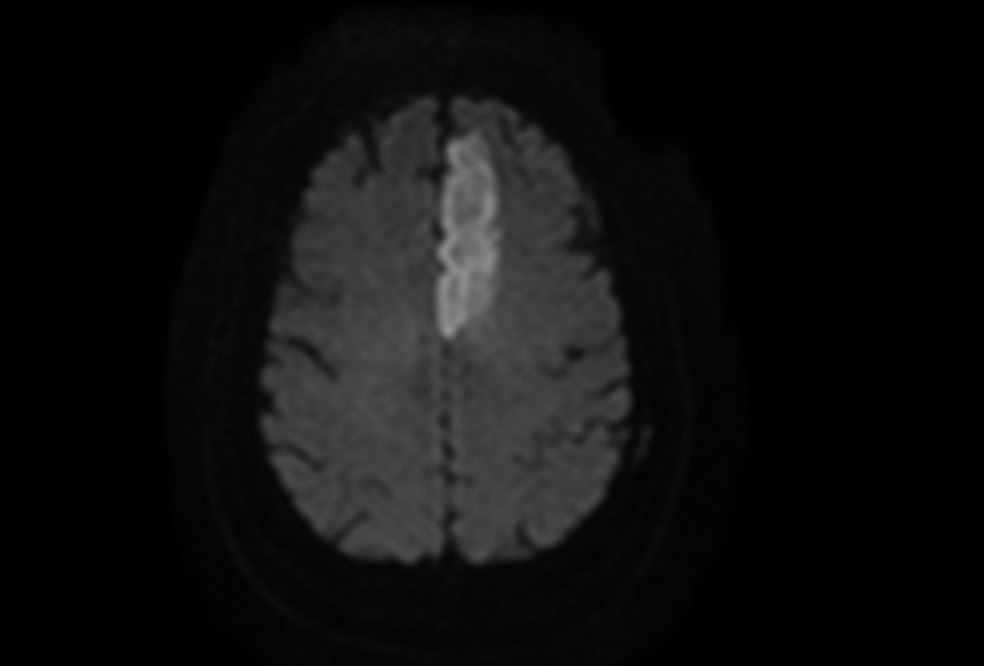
MRI DWI (left) demonstrating left ACA territory infarction. MRI, magnetic resonance imaging; ACA, anterior cerebral artery; DWI, diffusion-weighted imaging
